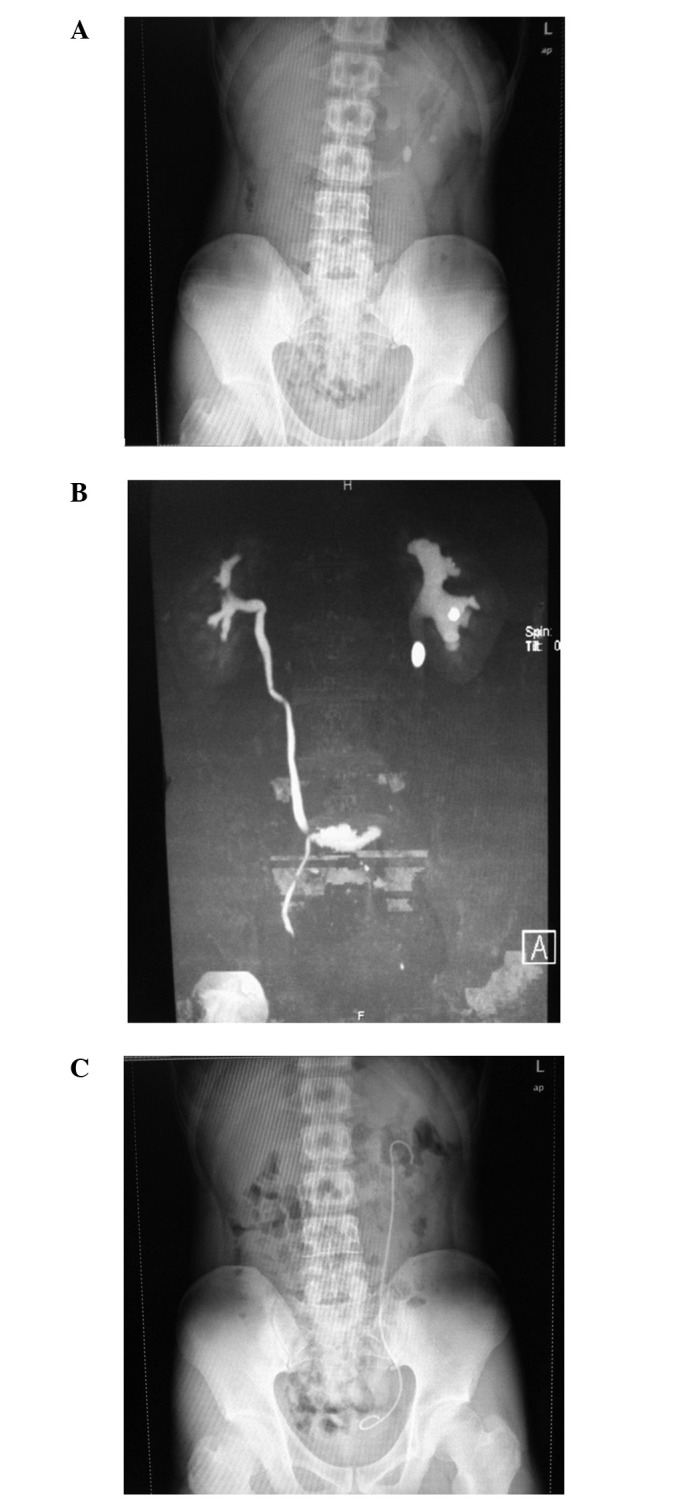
(A) Preoperative kidney, ureter and bladder (KUB) radiograph of Case 3, showing a stone in the upper left ureter (diameter 1.7 cm) and a stone in the left middle calyx (diameter 0.8 cm). (B) Preoperative computed tomography urography (CTU) scan of Case 3, showing a stone in the upper left ureter (diameter 1.7 cm) and a stone in the left middle calyx (diameter 0.8 cm). (C) KUB radiograph of Case 3 at day 1 postoperatively, indicating that the two stones were completely removed.
